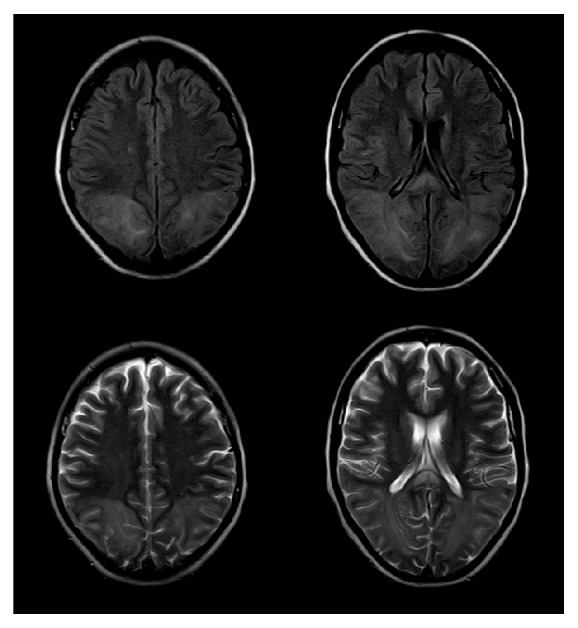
Bilateral symmetrical bright T2 and FLAIR white matter intensity involving the posterior parietal sections of both cerebral hemispheres, as well as the splenial segment of the corpus callosum.
